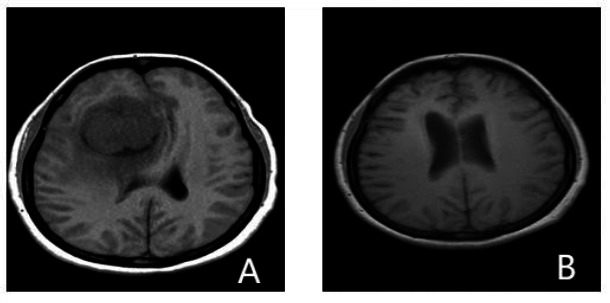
(A) Axial view of the preoperative T1-weighted MRI; (B) Axial view of the MRI performed at the eight-month postoperative follow-up.
